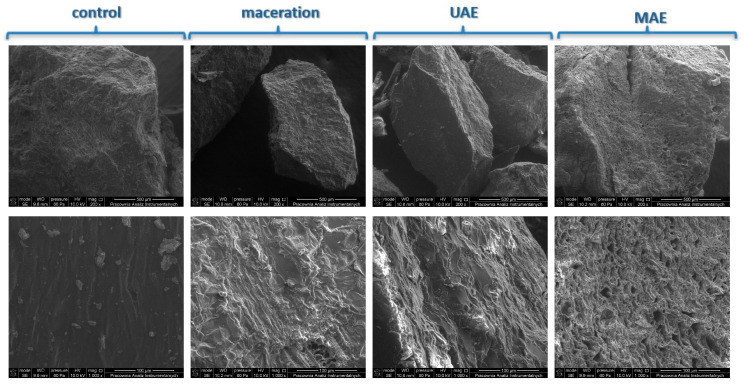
Representative SEM images of rose hip material after extraction by maceration, ultrasound-assisted extraction (UAE), and microwave-assisted extraction (MAE).
